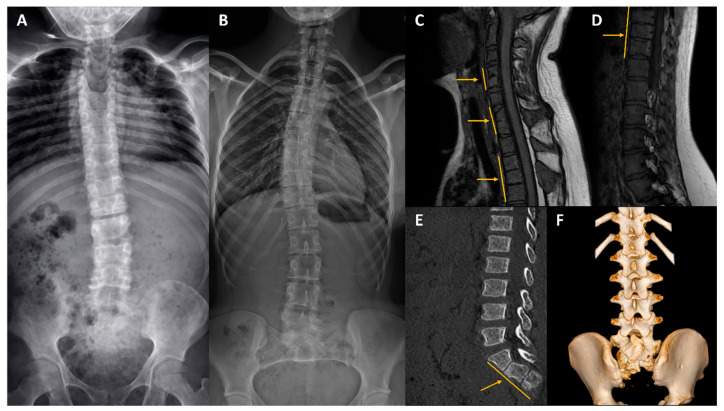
Representative imaging findings of congenital vertebral malformations in patients 2, 4, and 5. Standing anteroposterior spine radiograph, showing spinal deformity with block vertebrae (A). Standing anteroposterior radiograph demonstrating scoliosis with vertebral rotation (B). Sagittal MRI images showing multilevel congenital block vertebrae (arrows/lines) (C,D). Sagittal CT reconstruction (E) and 3D volume-rendered CT (F) demonstrating a malformed, hypoplastic sacrum with only S1 and S2 segments, and partially developed S3 segment, and absent coccyx (arrow/line). (A): Patient 2; (B–D): Patient 4; (E,F): Patient 5. Detailed radiological characteristics are provided in Table 3.
